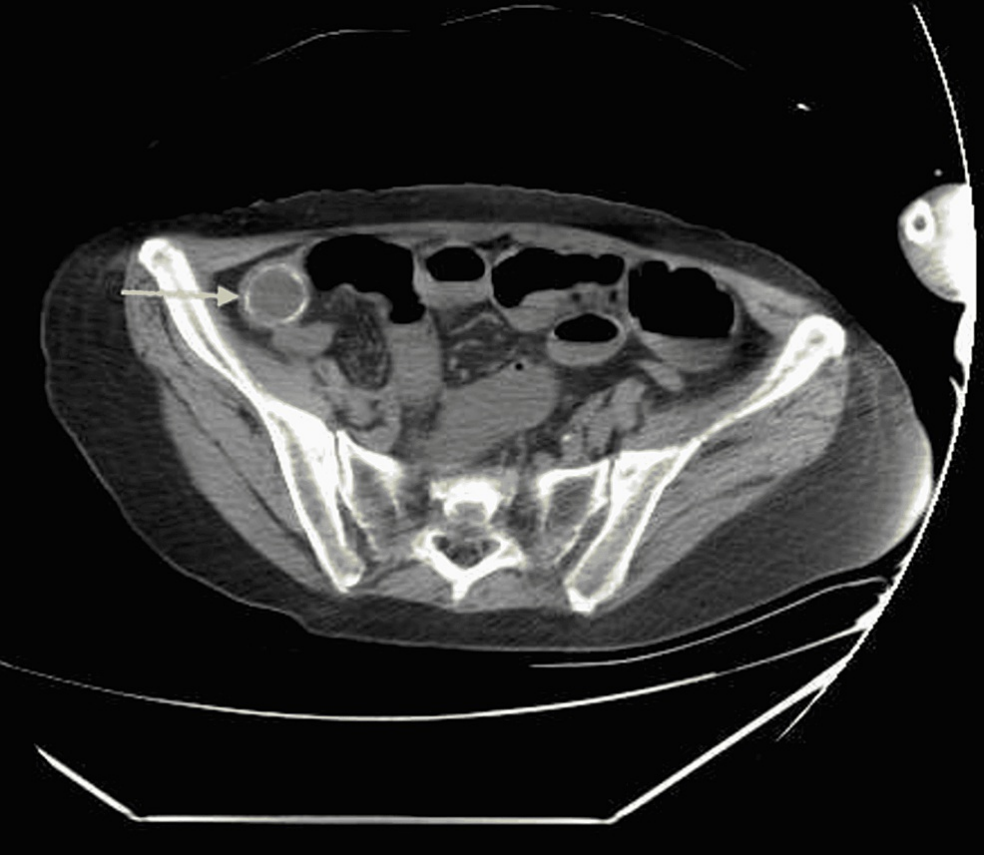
Axial view of abdominal computed tomography Gallstone in the distal ileum.
